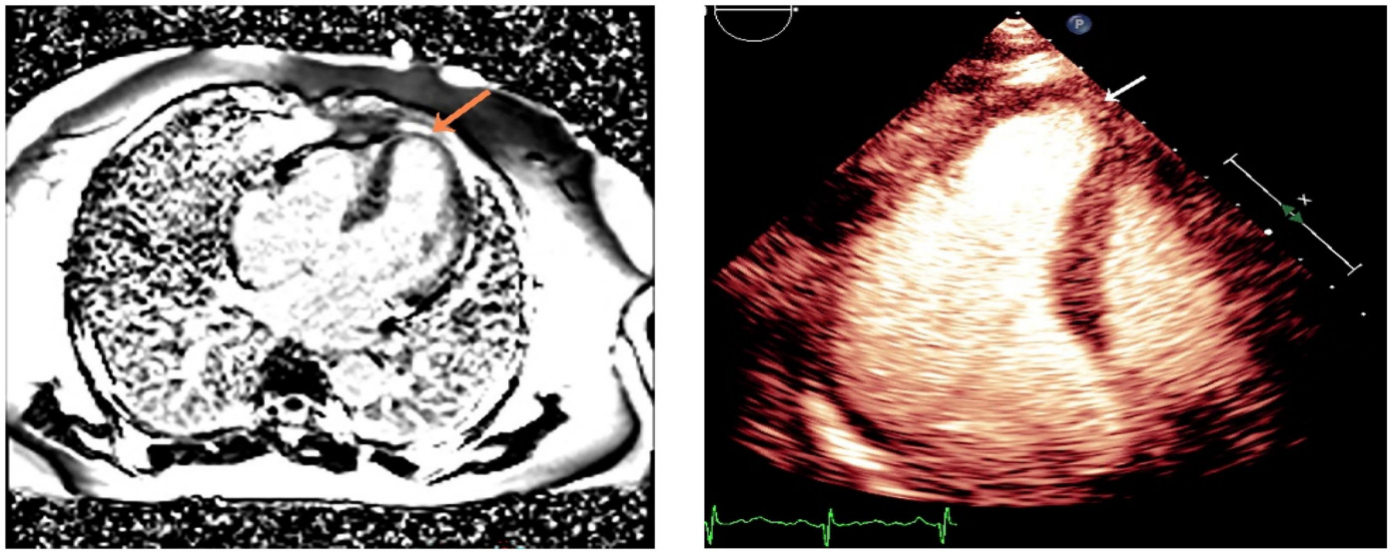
Three months later, MRI and MCE revealed the disappearance of the apical thrombus, with MCE showing significant improvement in myocardial perfusion. The orange arrow indicates the disappearance of the thrombus,while the white arrow highlights indicates the improved myocardial perfusion.
